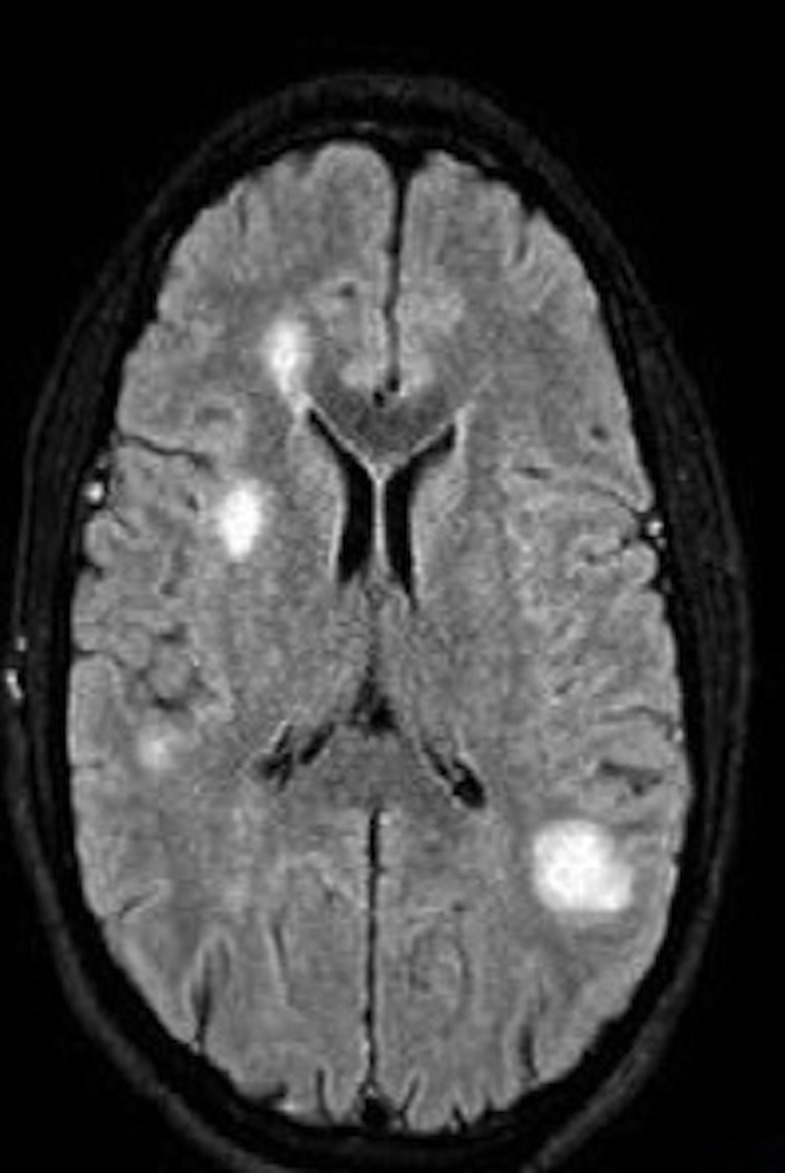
MRI after antimicrobial therapy shows decreased size of the lesions.
